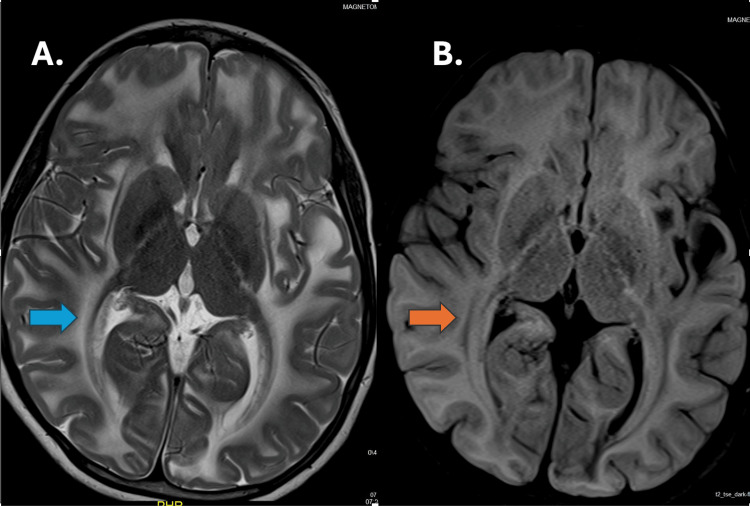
(A) Axial T2W 3-T and (B) axial FLAIR 3-T MRI images demonstrating bilateral, diffuse and symmetrical supratentorial white matter hyperintensities consistent with white matter edema (blue and orange arrows). T2W, T2 weighted; FLAIR, fluid-attenuated inversion recovery
